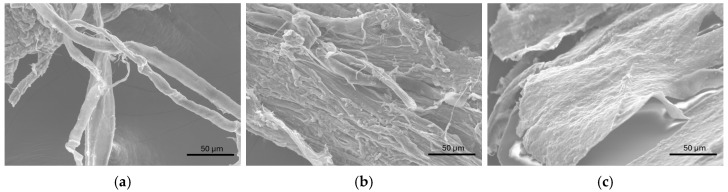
SEM images from the material collected from the surface of a Pt electrode in the study of complexes 25 (a,b) and 27 (c) in the presence of CT-DNA.
